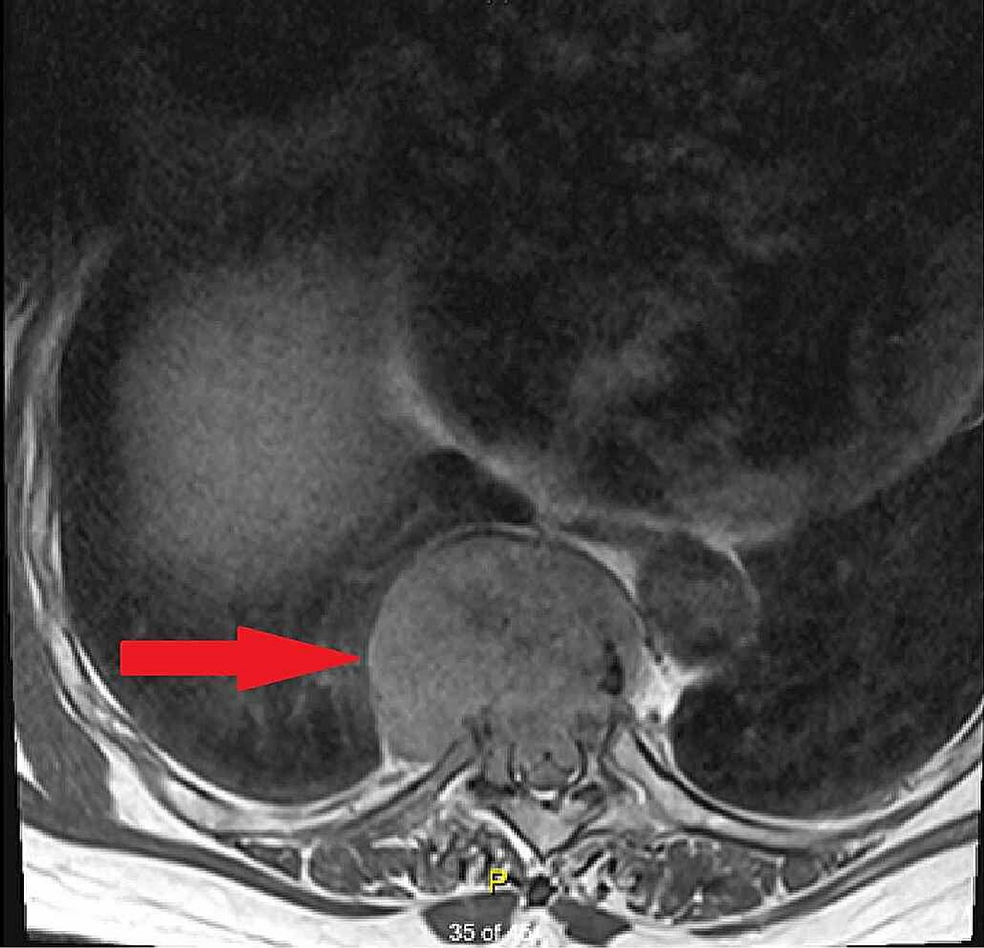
Plasmacytoma at the level of T8. Transverse view of thoracic spine via T2 MRI. Red arrow denoting the plasmacytoma within the central nervous system causing spinal cord compression
